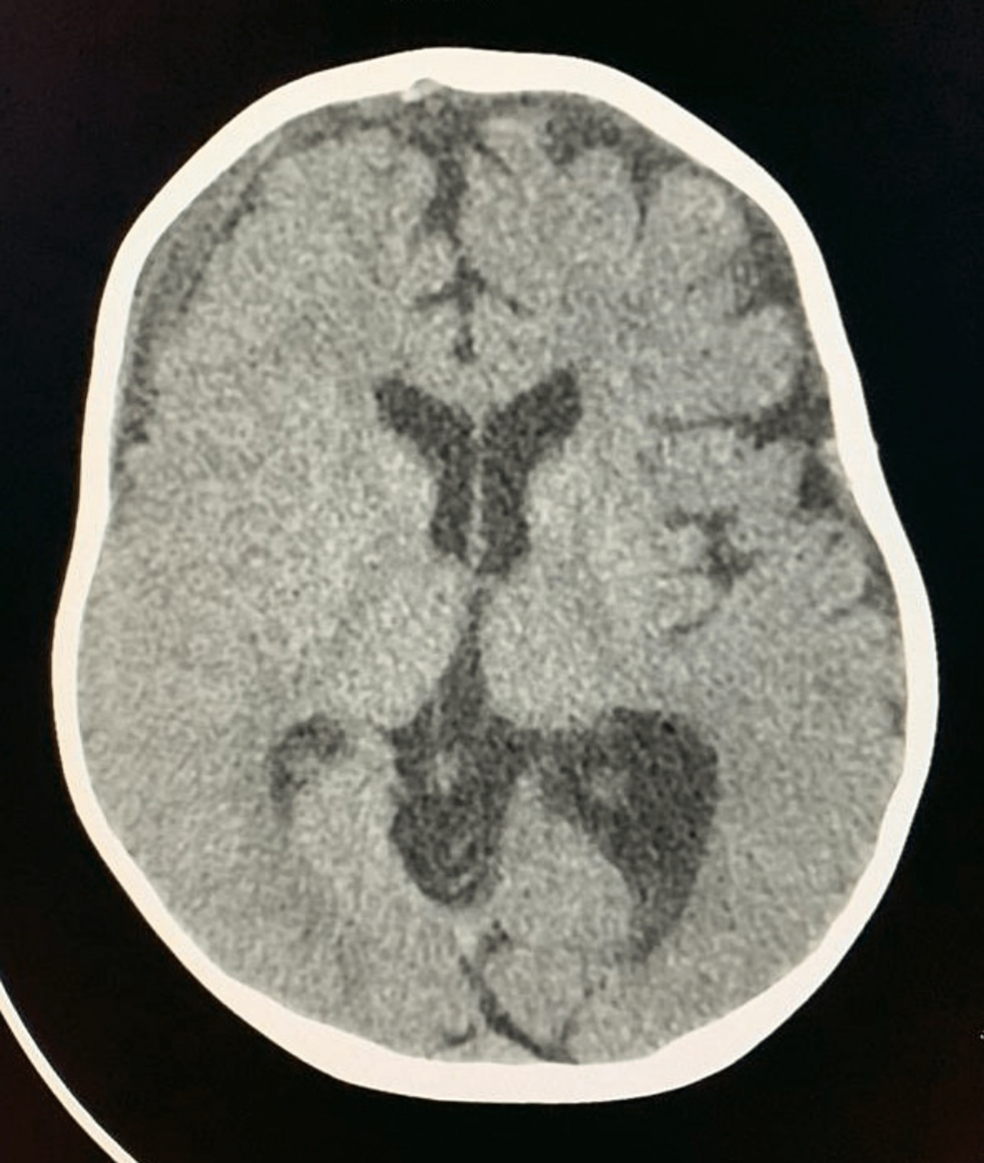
Axial section of brain CT (post evacuation of subdural hematoma) with no mass effect.
